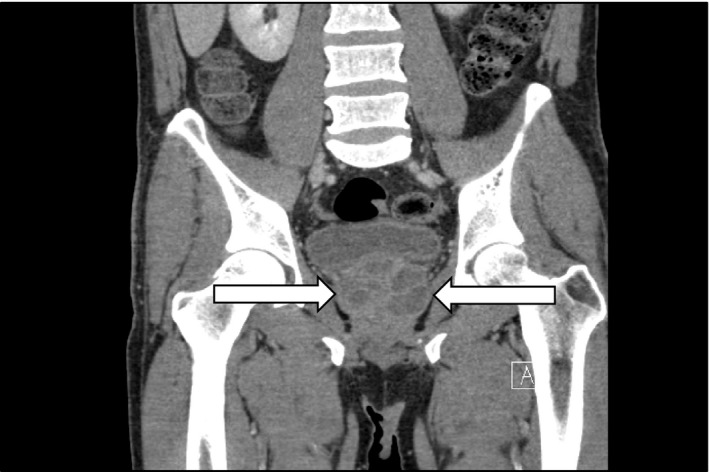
CT Abdomen Pelvis with a large multi‐septated prostate abscess
